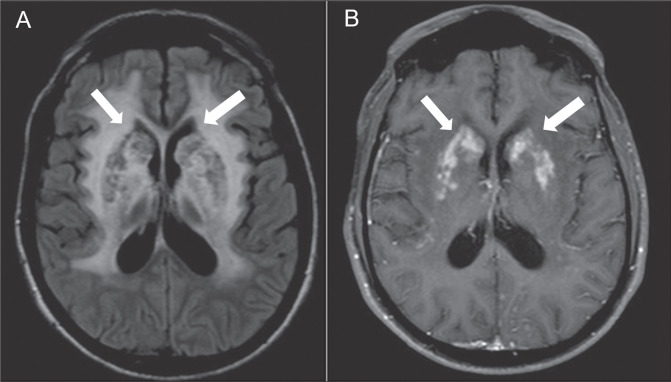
MRI scans of a patient with C-IRIS. Fluid-attenuated inversion
